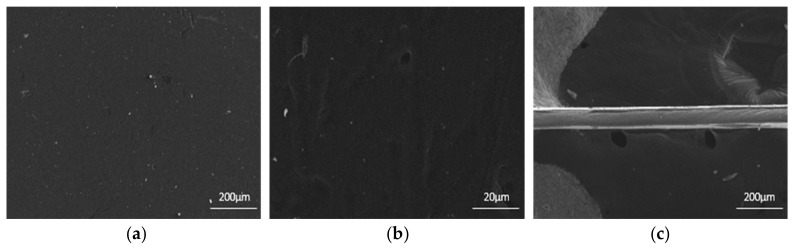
SEM images of CTA-100-BTS PIMs (30% CTA, 30% Aliquat 336, 40% BTS *w*/*w*): surface (a,b) and cross-section (c).
